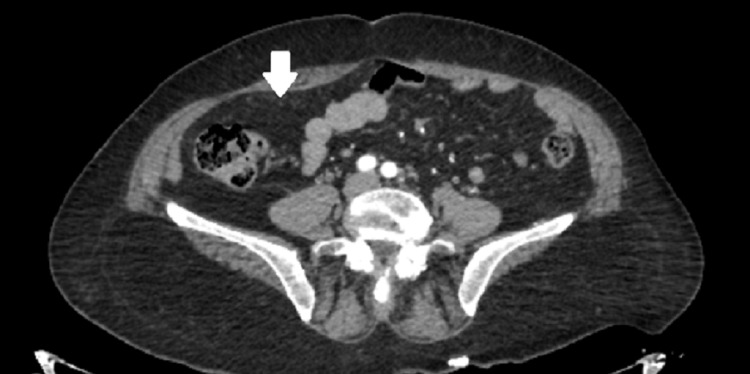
CT imaging on the first month of follow-up (the old hematoma area is marked with an arrow) CT: computed tomography
